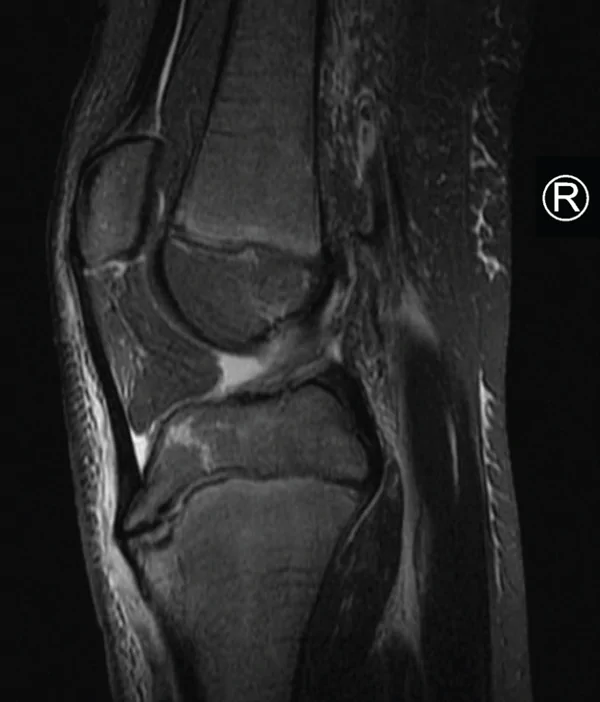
Right knee sagittal T2 MRI.
